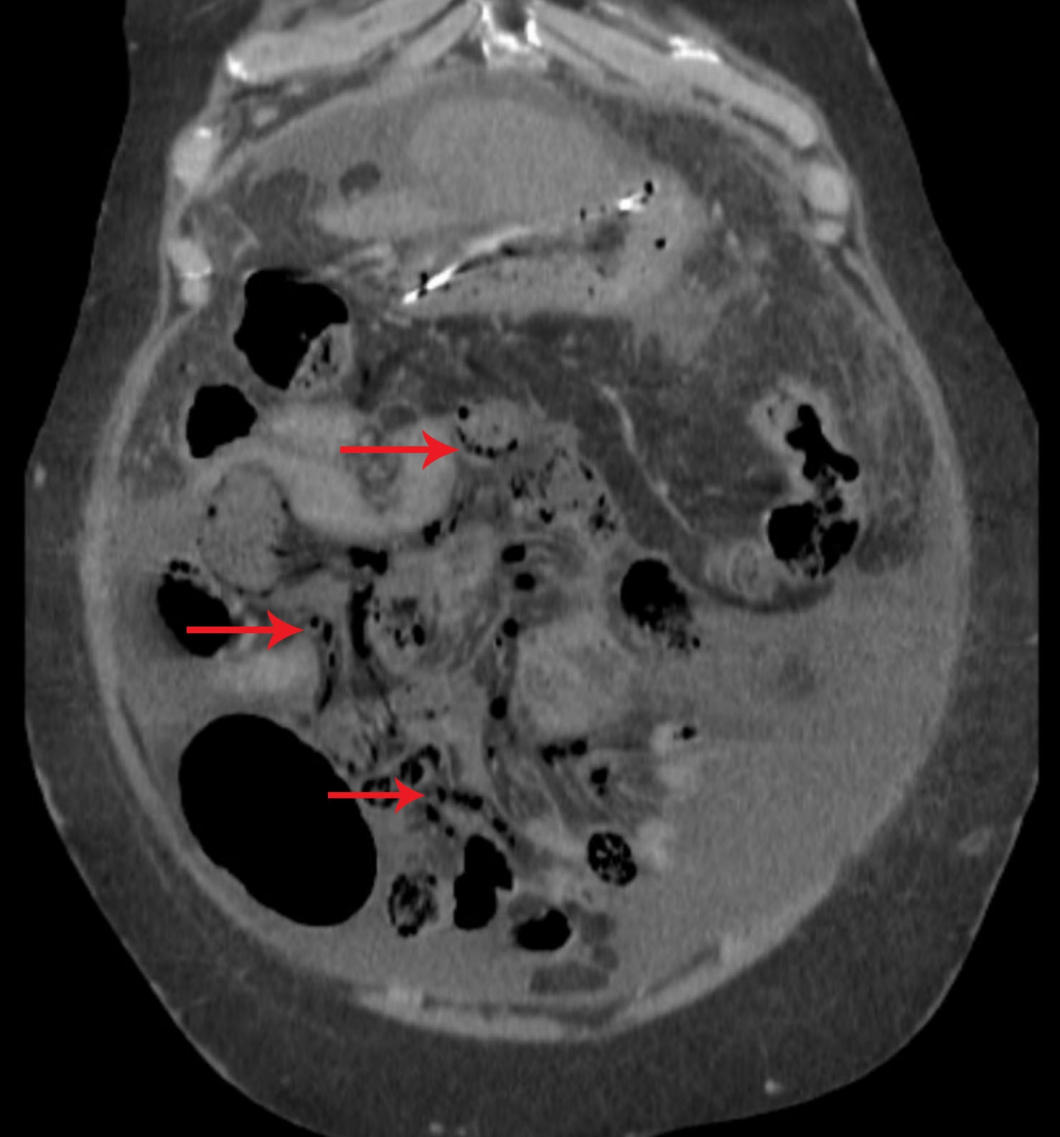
CT abdomen and pelvis demonstrating extensive mesenteric gas (red arrows) concerning for bowel ischemia in the setting of cryptococcal peritonitis induced abdominal compartment syndrome in a patient with advanced liver disease CT: computed tomography
